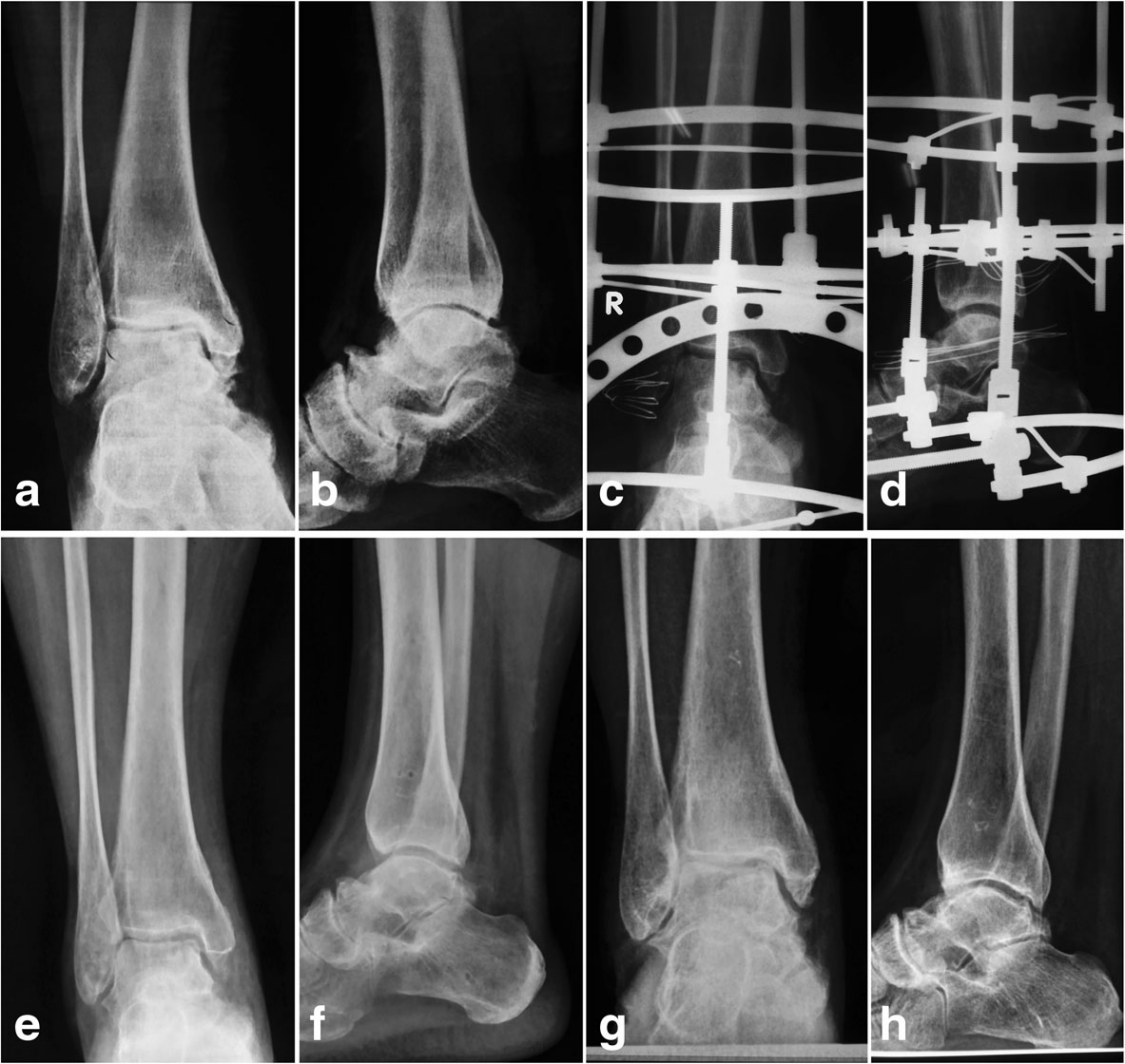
Preoperative X-ray of a 51-year-old female showed a van Dijk stage II osteoarthritis of the right ankle joint; the preoperative ankle joint space distance was 2.3 mm (a, b). The patient was treated with open debridement and distraction arthroplasty; the postoperative X-ray showed clear joint space and was enlarged to 6.0 mm (c, d). The 1-year follow-up X-ray showed clear joint space and diminishing of subchondral sclerosis, and the joint space was 4.6 mm (e, f). The 3-year follow-up X-ray showed that the joint space is still clear and with a distance of 3.8 mm (g, h), and the patient was pain-free
